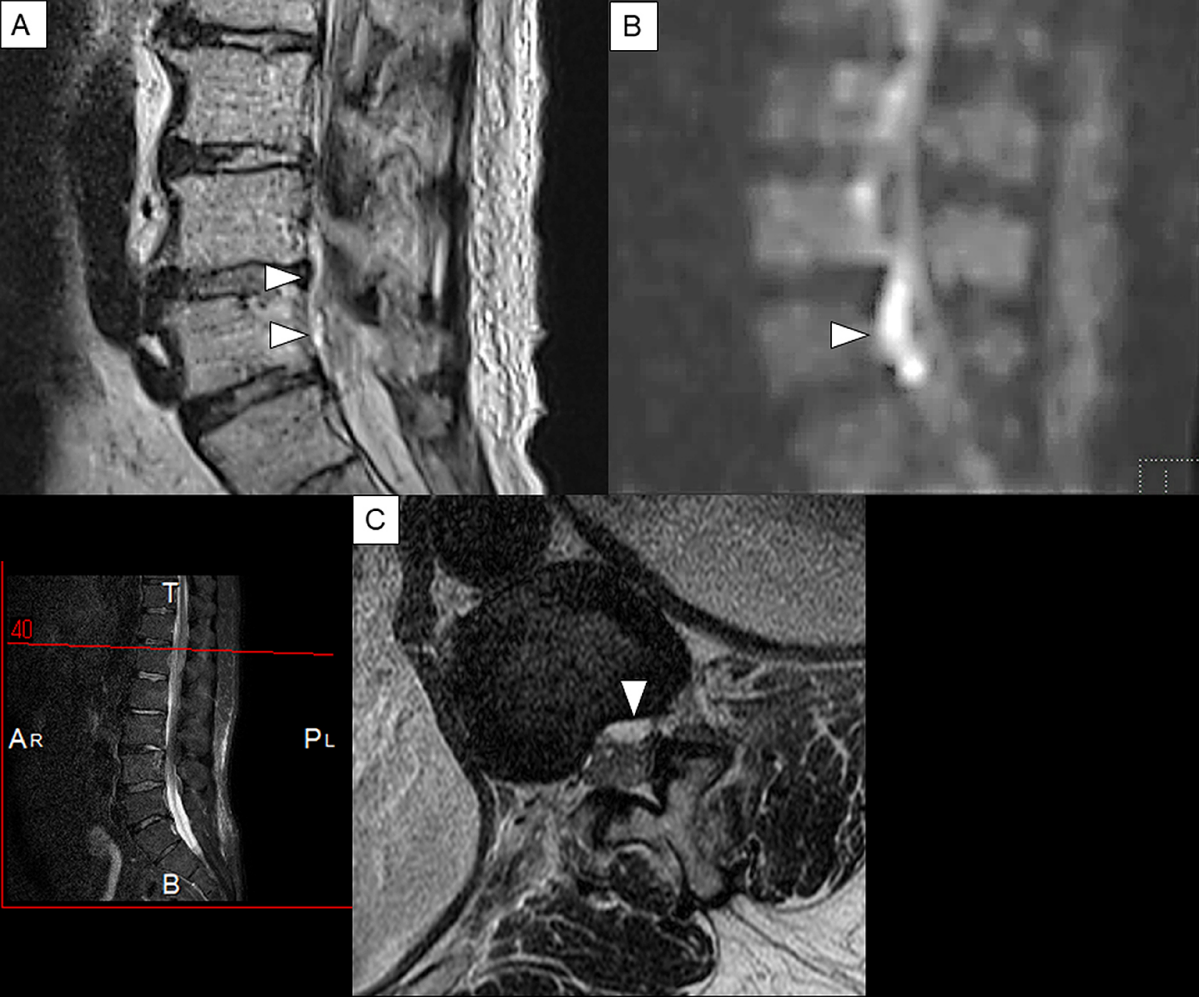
Sagittal T2-weighted and Gd-enhanced MRI of the lumbar spine in case2. (A): Sagittal view of T2-weighted MRI.(B): Sagittal view of diffusion-weighted image. (C): Axial view of T2-weighted MRI. A linear lesion that shows high intensity and appears to be an epidural abscess that is found on the dorsal side of the vertebral body (A). The same lesion shows a strong high signal area in the diffusion-weighted image (B). This finding is considered to reflect the decrease in diffusion of water molecules in the abscess lumen and is characteristic findings of abscess. The axial view confirms anterior SEA that shows high signal intensity on the dorsal side of the vertebral body at the level of L1 (C). Arrowheads indicate SEA.
